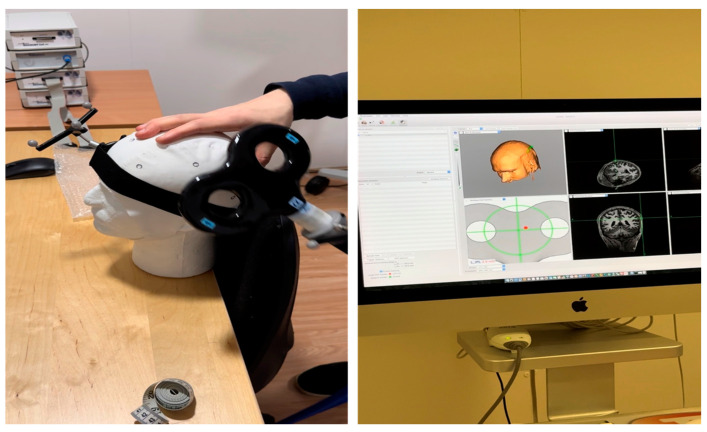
Example of the MRI-guided placement of a magnetic coil over the left IPL (with the kindness of Prof. Dr. Neil Basu from the University of Glasgow).
